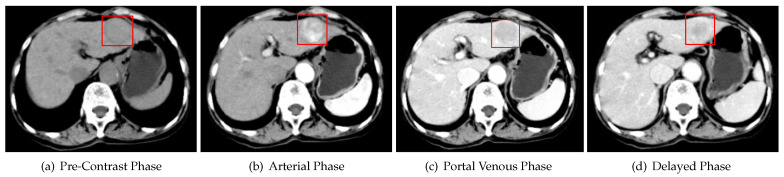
Four-phase image of CT images. Lesion area is labeled by the red box: (a) shows the pre-contrast phase image without injecting intravenous contrast media; (b) shows the lesion areas tend to enhance more strongly than background liver during late arterial phase imaging (APHE); (c) shows the liver continues to enhance, and the lack of portal venous blood supply to HCCs results in the characteristic washout in the portal venous phase (WO); (d) shows the hypodense appearance of HCC in the delayed phases (WO).
